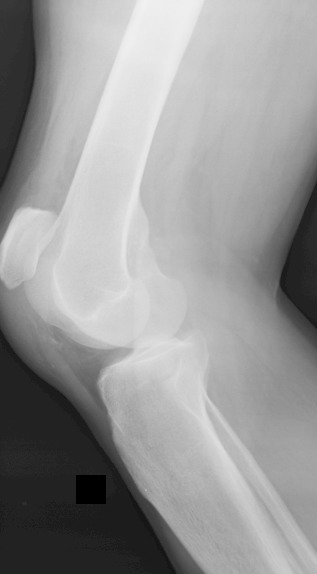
Radiographie de profil du genou gauche objectivant la patella alta
